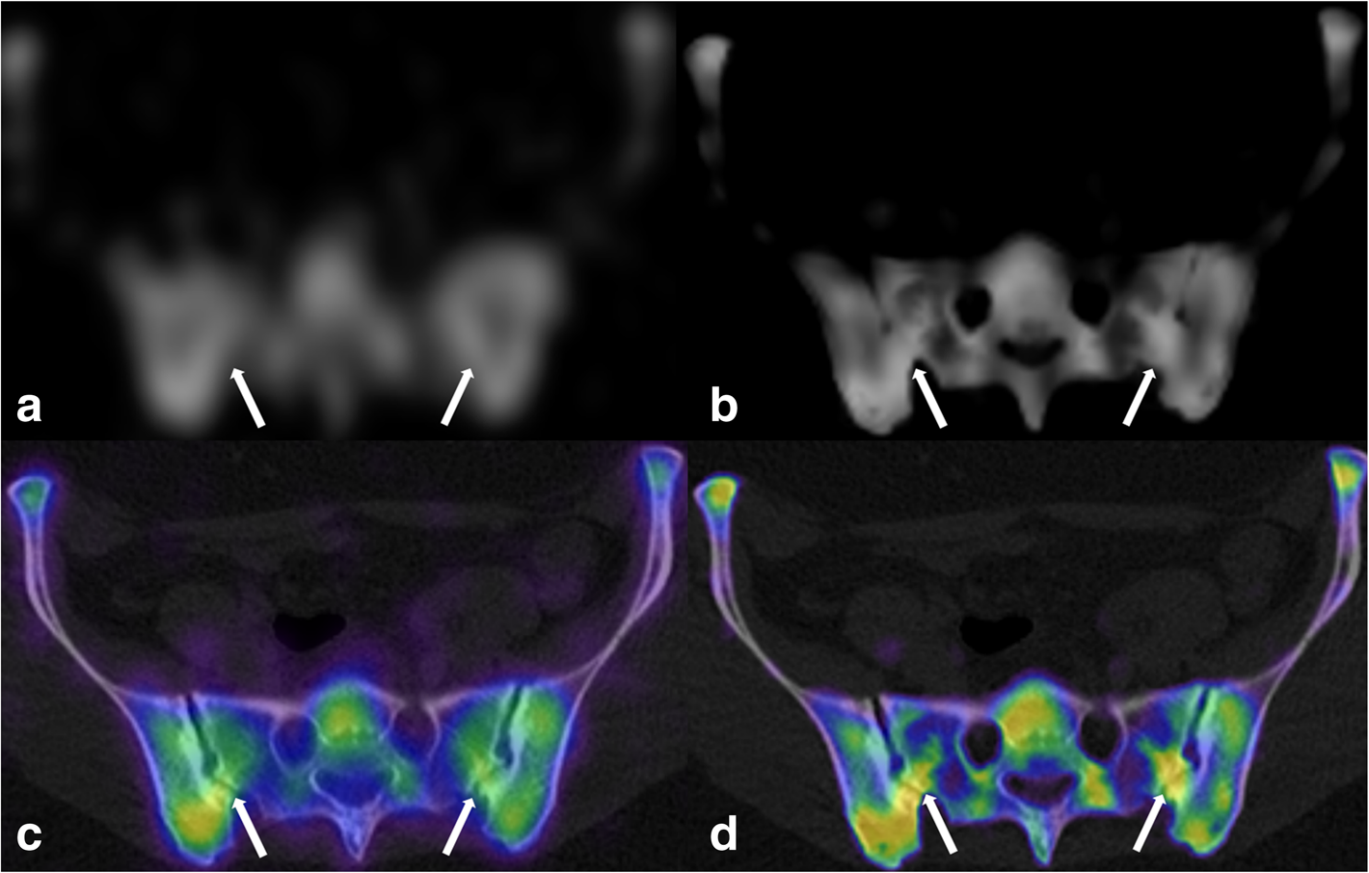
Osteoarthritis Left Posterior Sacroiliac Joints. Detailed Legend: 35 yr. old female with lower back pain and degenerative changes in posterior synovial sacroiliac joints (white arrows). Single transaxial slice (all same slice): a SPECT, b xSPECT, c SPECT-CT, and d xSPECT-CT. Note the difficulty in identifying any abnormality in the SPECT slice (a)
